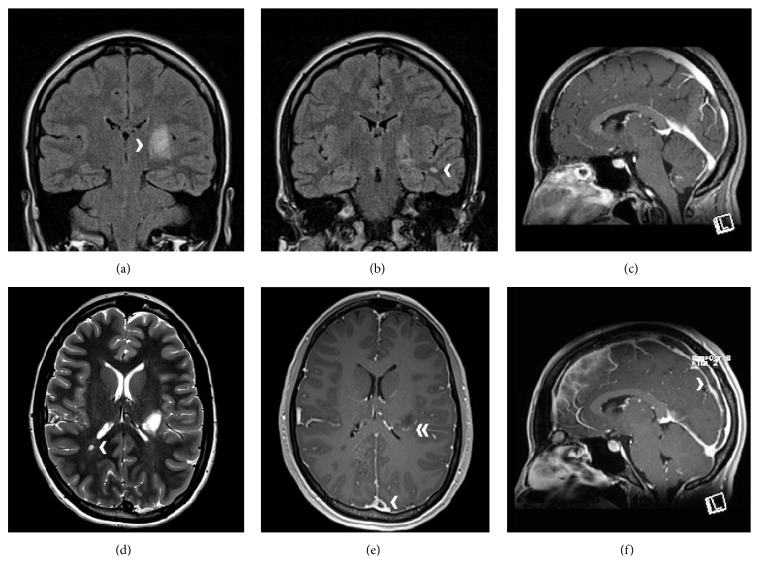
The magnetic resonance imaging of the patient reveals a large hyperintense lesion on FLAIR (a) and T2 (d) in the posterior limb of the left-sided internal capsule, probably extending to the thalamus. The other supratentorial lesions are much smaller (in the right occipital (d) and left temporal white matter ((b) FLAIR)). In the initial imaging the blood flow in the sagittal sinus was free ((c) gadolinium (Gd) enhanced T1w). 8 days later the white matter lesions were unchanged ((d) T2w, arrowhead pointing out a small lesion behind the occipital horn of the right ventricle) and some lesions were still Gd enhancing ((e) double arrowhead, Gd enhanced T1w). But now a thrombosis of the superior sagittal sinus can be seen ((e), (f) arrowheads, Gd enhanced T1w).
